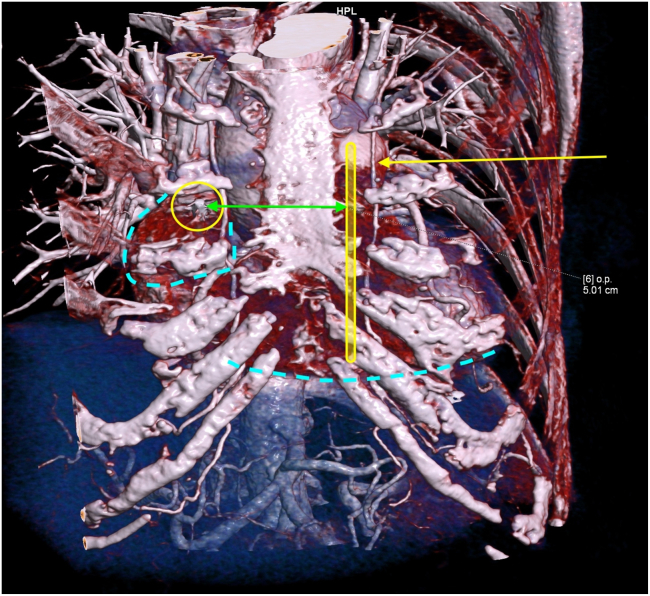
3-dimensional reconstruction of the chest computed tomography. *Yellow rectangle:* Planned Epsila EV lead placement. *Yellow circle:* Atrial lead tip of the transvenous dual-chamber permanent pacemaker. *Yellow arrow:* Left internal mammary artery. *Green arrow:* Distance between the atrial lead tip and the Epsila EV lead, 5.01 cm. *Dotted blue lines:* Right atrial appendage over the right side and the inferior border of the right ventricle. EV = extravascular.
